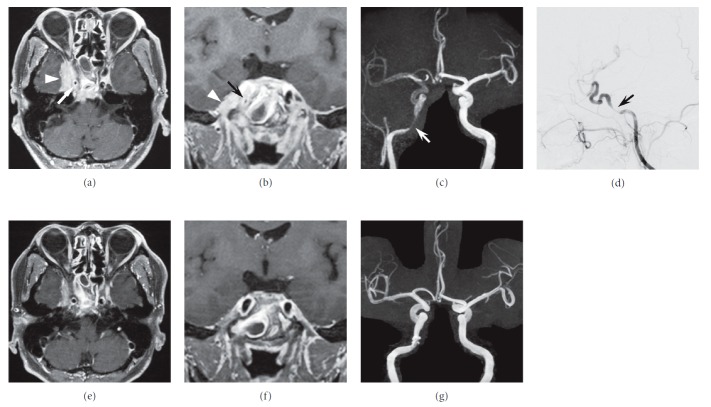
Imaging studies before immunosuppressive treatment (a–d) and 2 years after the initiation of the treatment (e–g). Gadolinium-enhanced T1-weighted MRI of the brain reveals thickening of enhanced dura mater extending from the cavernous sinus to the clivus on axial (a) and coronal (b) images (triangle). Narrowing at the C5 portion of the internal carotid artery (ICA) is apparent on axial (a) and coronal (b) MRI, MRA (c), and lateral cerebral angiography of the ICA (d) (arrow). Thickening of the dura mater and stenosis of the ICA improved on axial (e) and coronal (f) MRI and MRA (g).
